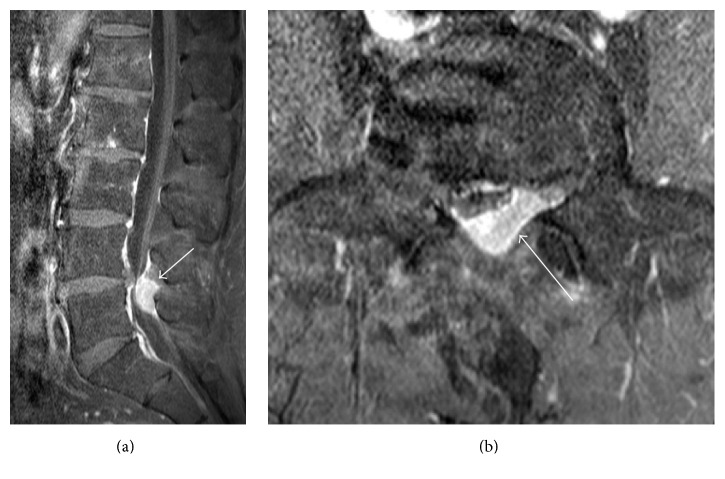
Sagittal (a) and axial (b) gadolinium-enhanced T1-weighted MR images with fat saturation show intense enhancement of solitary spinal epidural mass (arrow).
